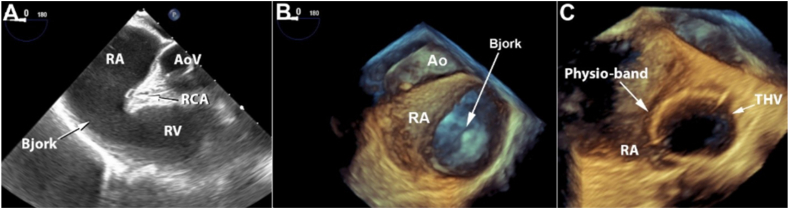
A) Intra-operative transesophageal echocardiography demonstrating the non-valve Bjork connection from the RA to the RV, the right coronary artery (RCA) is labeled as is the aortic valve (AoV). B) 3-D reconstruction of multiplanar TEE imaging showing the elliptical shape of the large Bjork connection as seen from the RA view. C) TEE 3-D reconstruction following hybrid Physio-band and THV placement as seen from the RA view.
